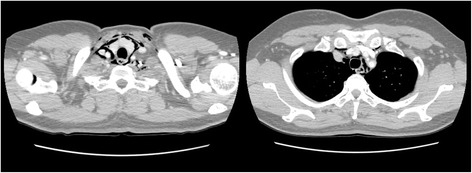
Chest computed tomography revealing emphysema extending to the mediastinum
